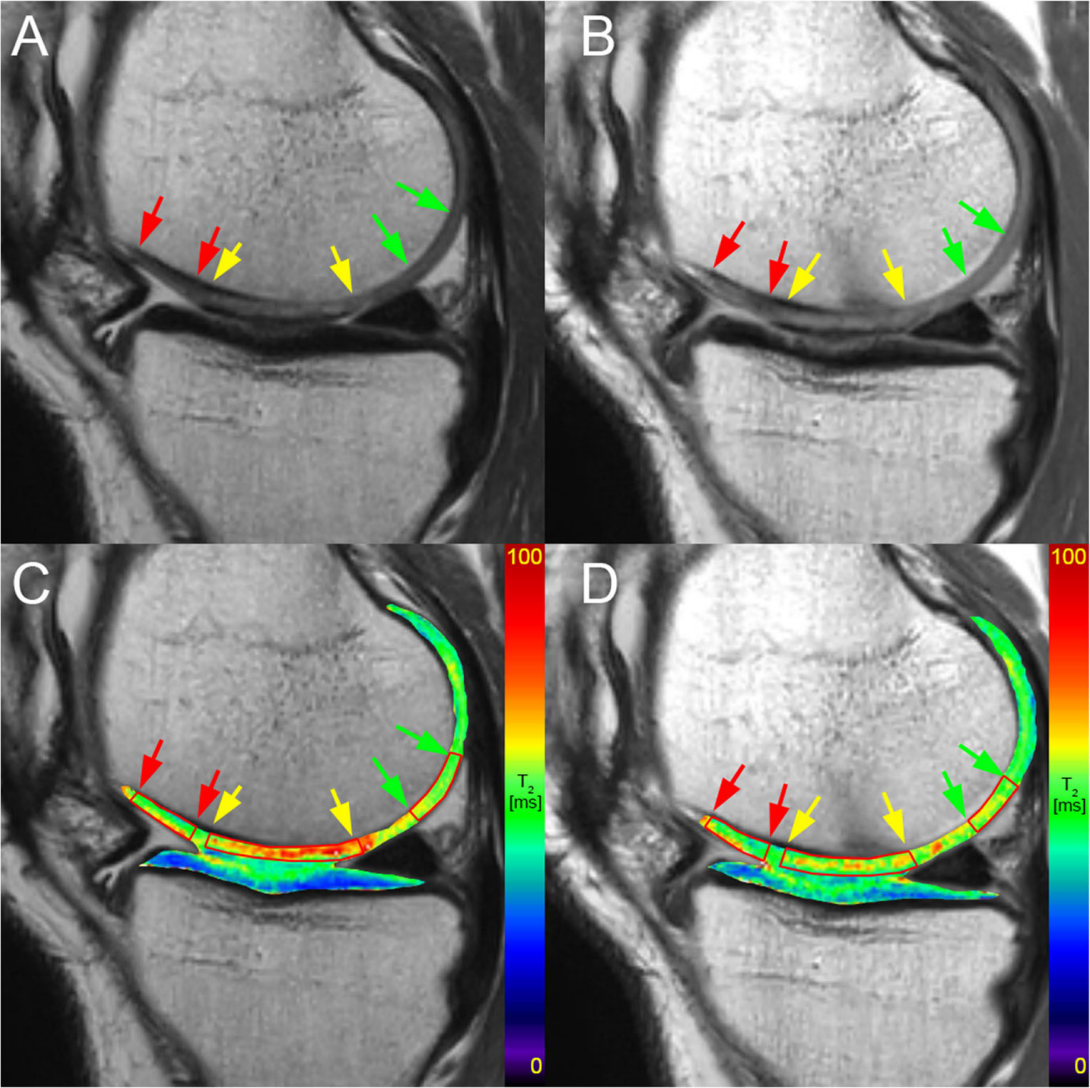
ROI selection on T_2_-weighted MR images at 12 months and 24 months (A and B, respectively). ROIs were transferred onto the T_2_ maps (red delineation) at 12 and 24 months (C and D, respectively). Yellow arrows point toward the lesion site boundaries, red arrows point toward the adjacent tissue boundaries and green arrows point toward the reference tissue boundaries
